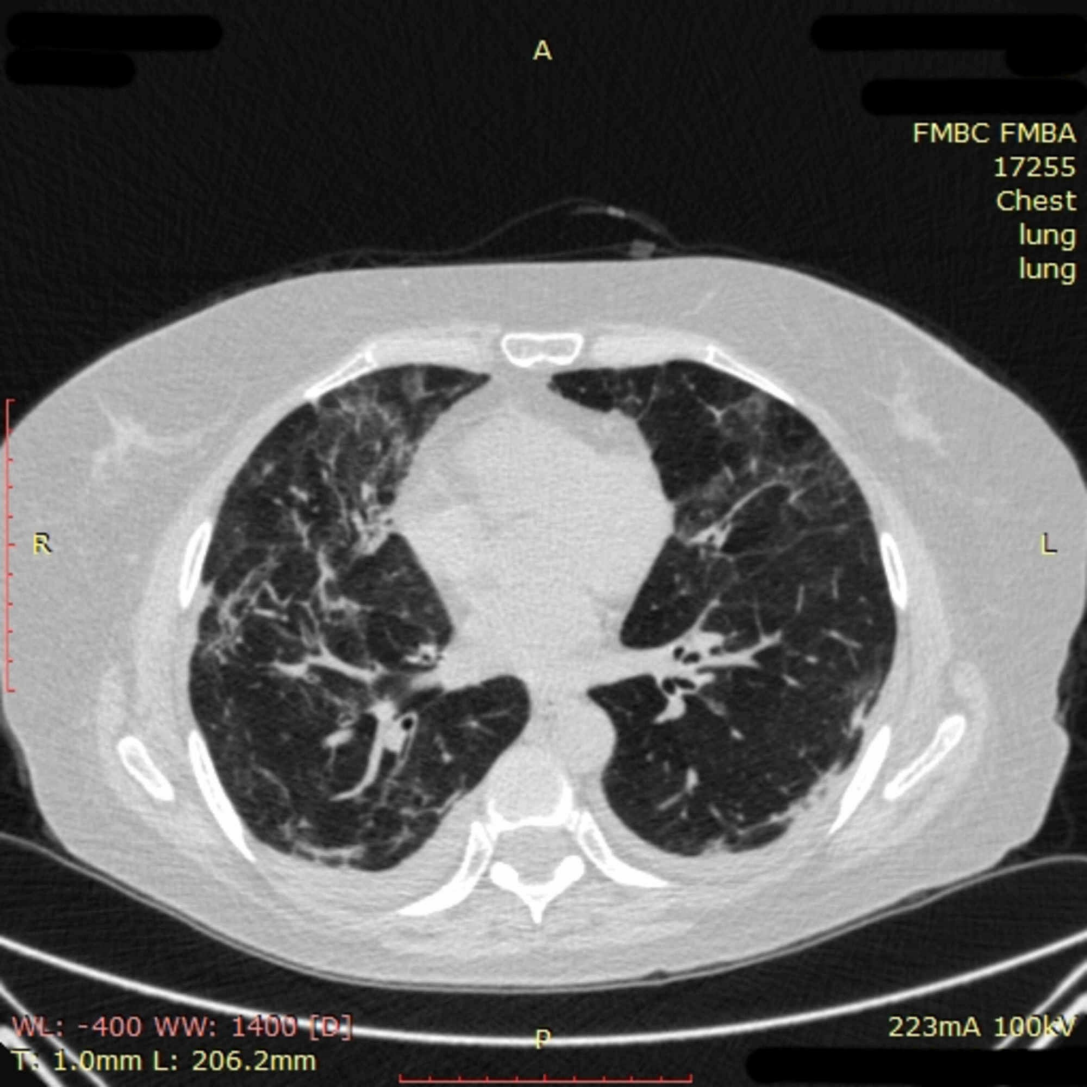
Chest CT imaging of patient 1 on day 22 Chest CT on day 22 showed that opacification in the left lung had mostly resolved, and the area and density of obfuscation in the right lung had clearly decreased CT: computed tomography
